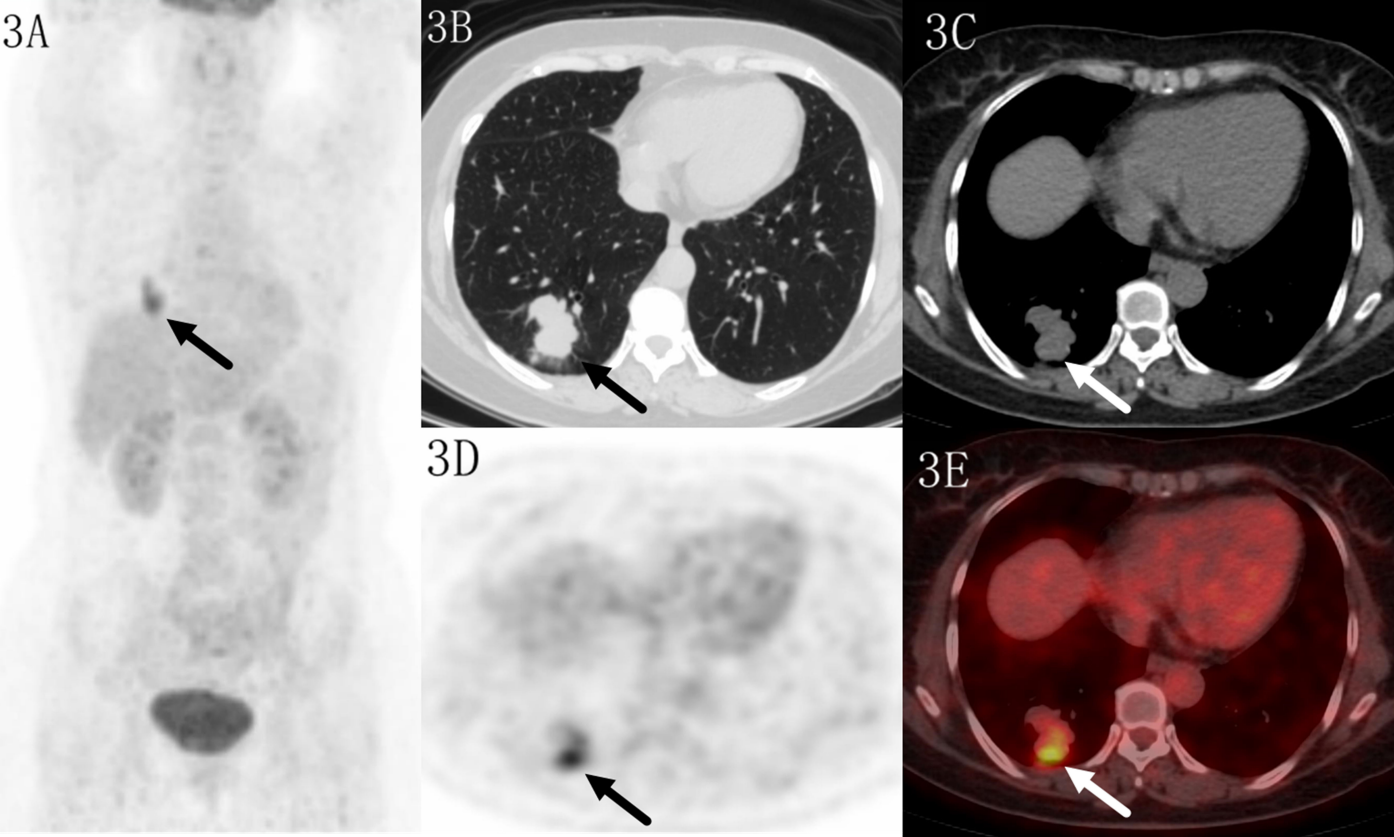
Images of a 52-year-old female patient with tuberculosis. PET MIP (A) showed a mass in the right lower lobe. The lobular lesion located in peripheral site, with a SUVmax of 6.6 on transaxial CT (B, C), PET (D) and fused (E) images. Higher uptake was seen in the distal region which indicated benign lesion.
